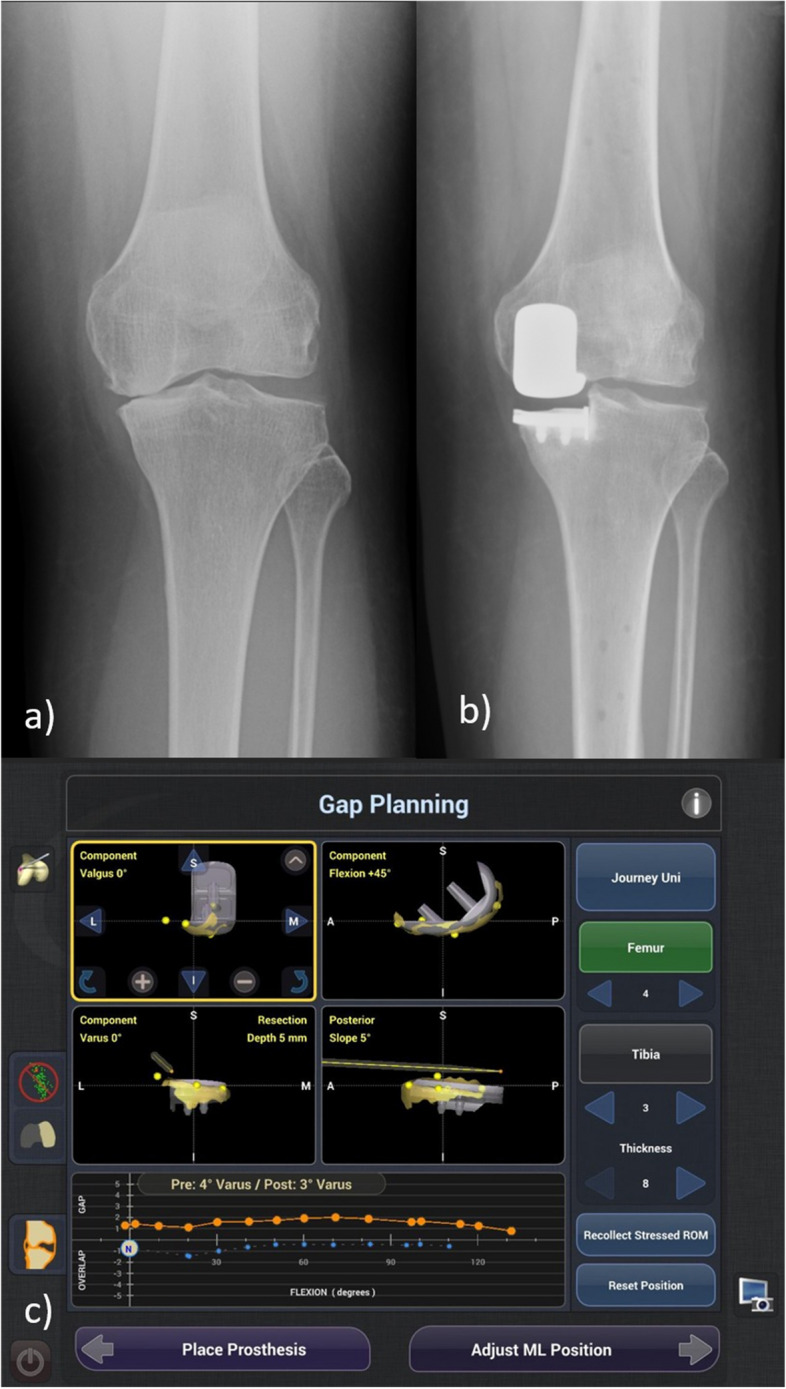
a Preoperative X-ray; b postoperative X-ray of R-UKA; c Navio image-free robotic system intraoperative planning
